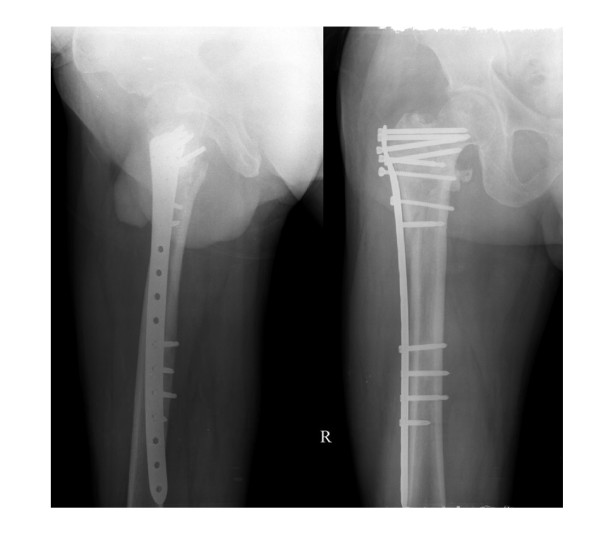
AP and lateral radiograph at 16 weeks post fixation showing union of the fractures.
